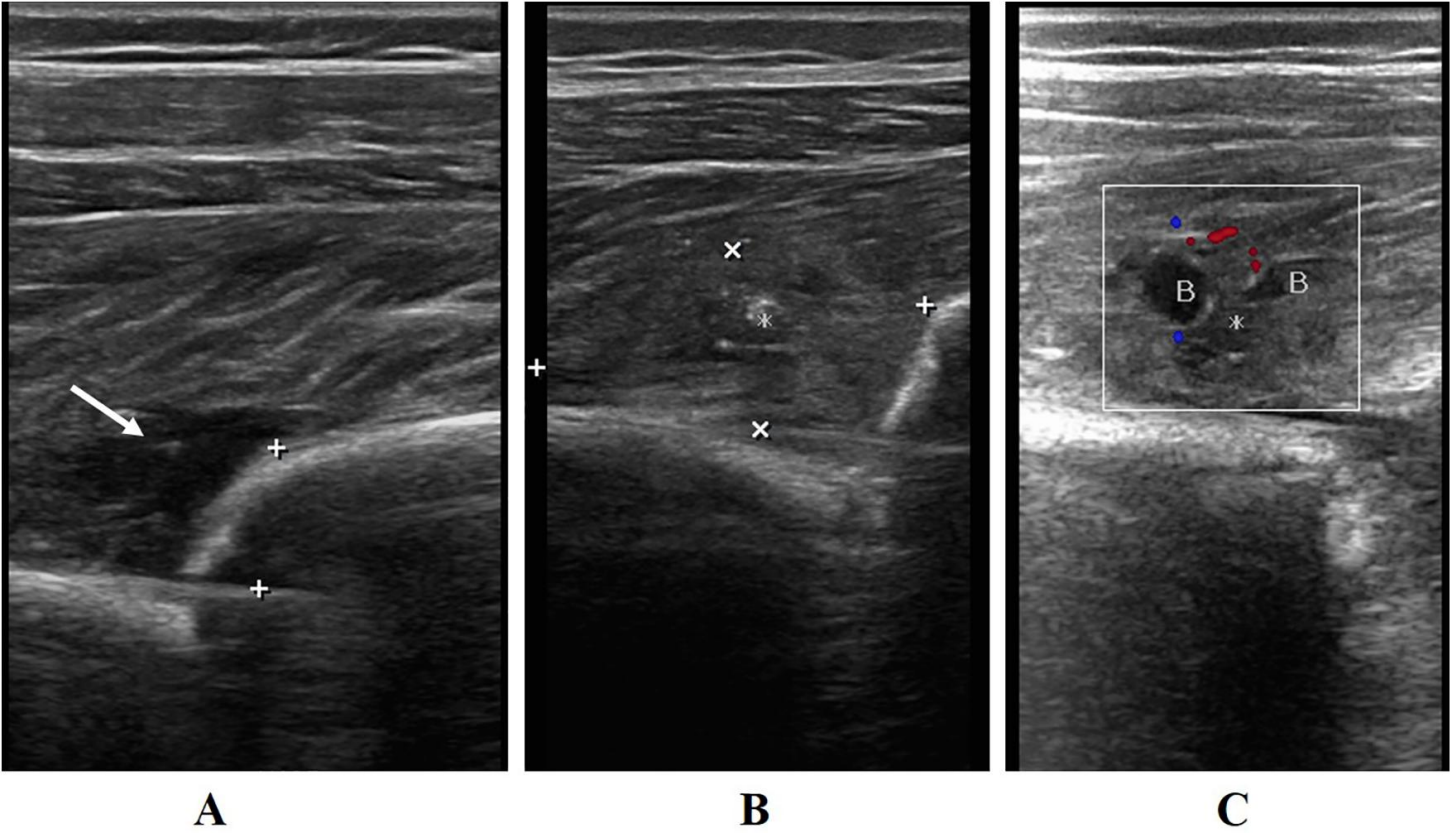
(A) longitudinal section of a 4-year-old child with a left middle femur fracture reveals a hematoma (white arrow) at the edge of the fracture (+) on ultrasound. (B) Longitudinal section of bone callus length (+) and thickness (×) on ultrasound 1 week after the injury. (C) Blood supply (B) to the bone callus (*) on Color Doppler ultrasound 1 week after the injury.
